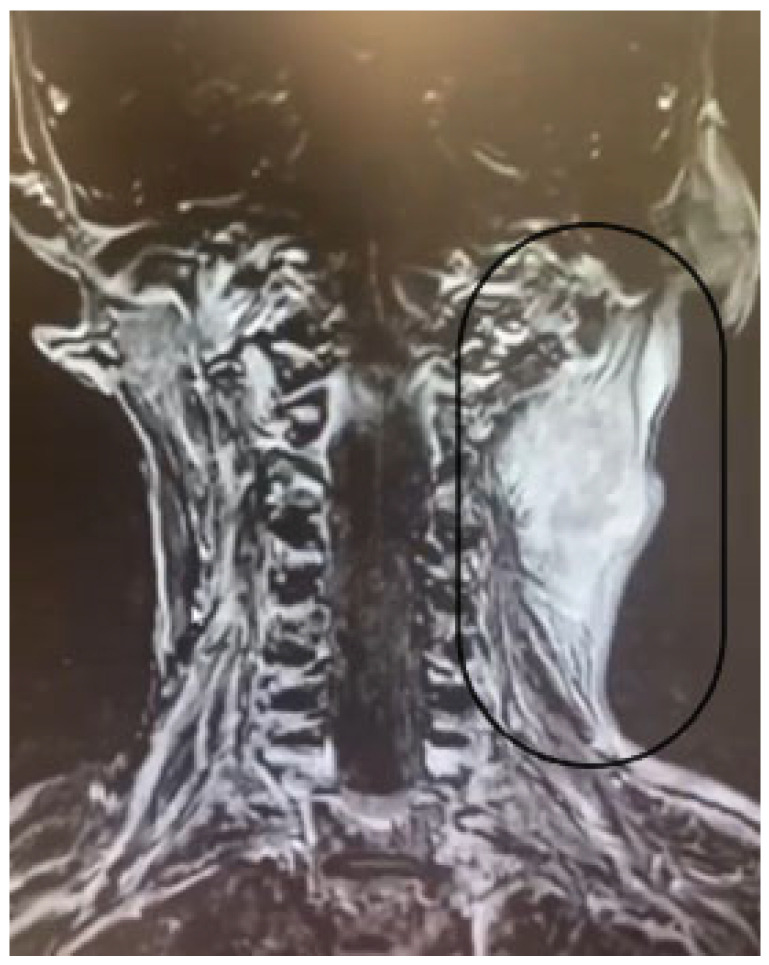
Cervical region MRI: cervical superior confluent lymph node on the left side, tangent to the jugular–carotid vascular bundle and the lower pole of the left parotid gland (circle).
